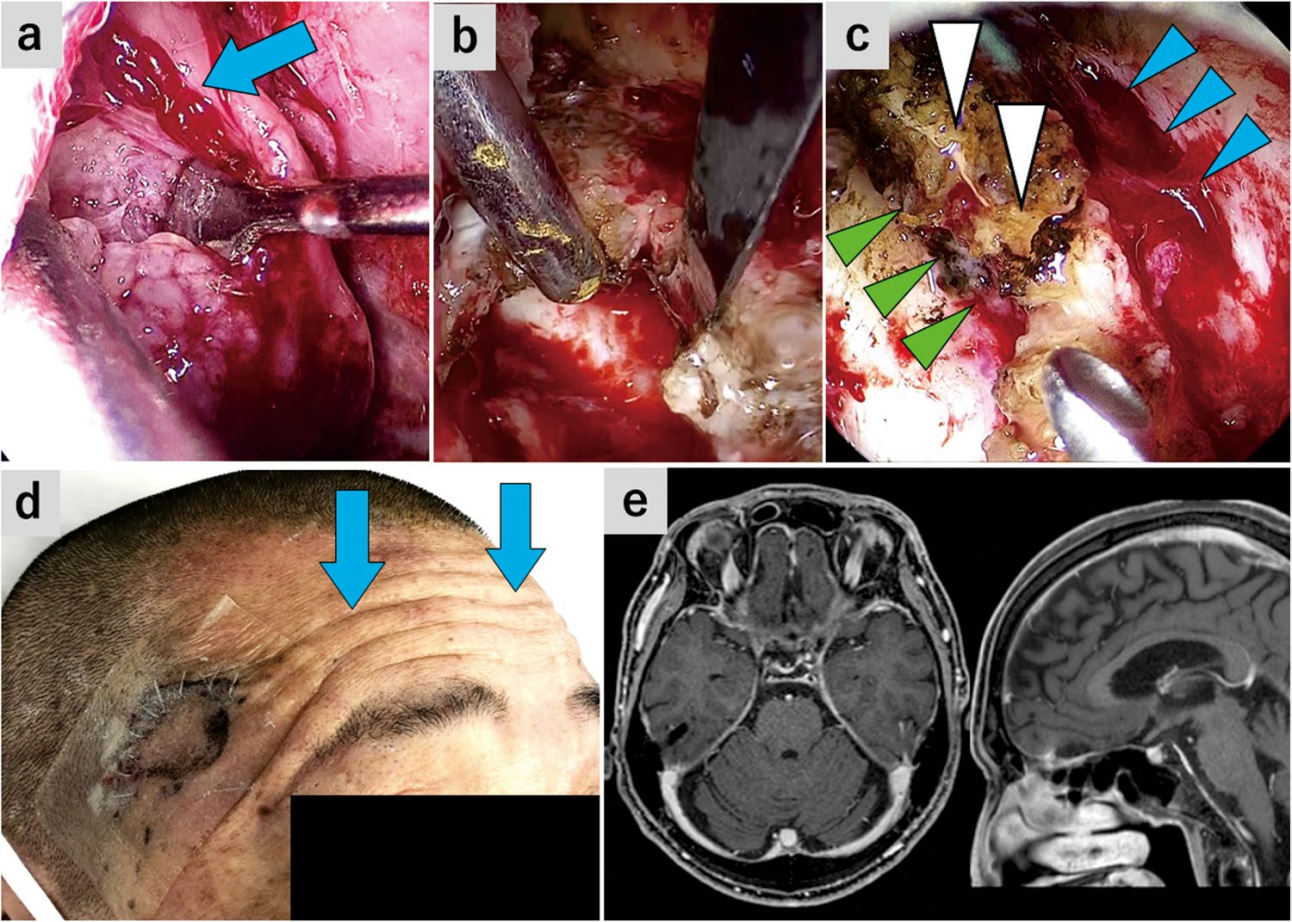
Case 2: Olfactory groove meningioma (a–c) The endoscope was inserted into the subfrontal space and the remnant tumor was resected. a The anterior part of the tumor involving the right olfactory tract was dissected. Blue arrow, right olfactory tract. b The posteroinferior edge of the falx is transected to remove the tumor invading the left olfactory groove. c The left side of the tumor was also excised. Blue arrowheads, right olfactory groove; white arrowheads, cut surface of the falx; green arrowheads, left olfactory groove. d The image immediately after surgery showing the wound location and normal frontalis muscle contraction. Blue arrows, Frontalis muscle contraction (e) Postoperative gadolinium-enhanced T1-weighted MRI
